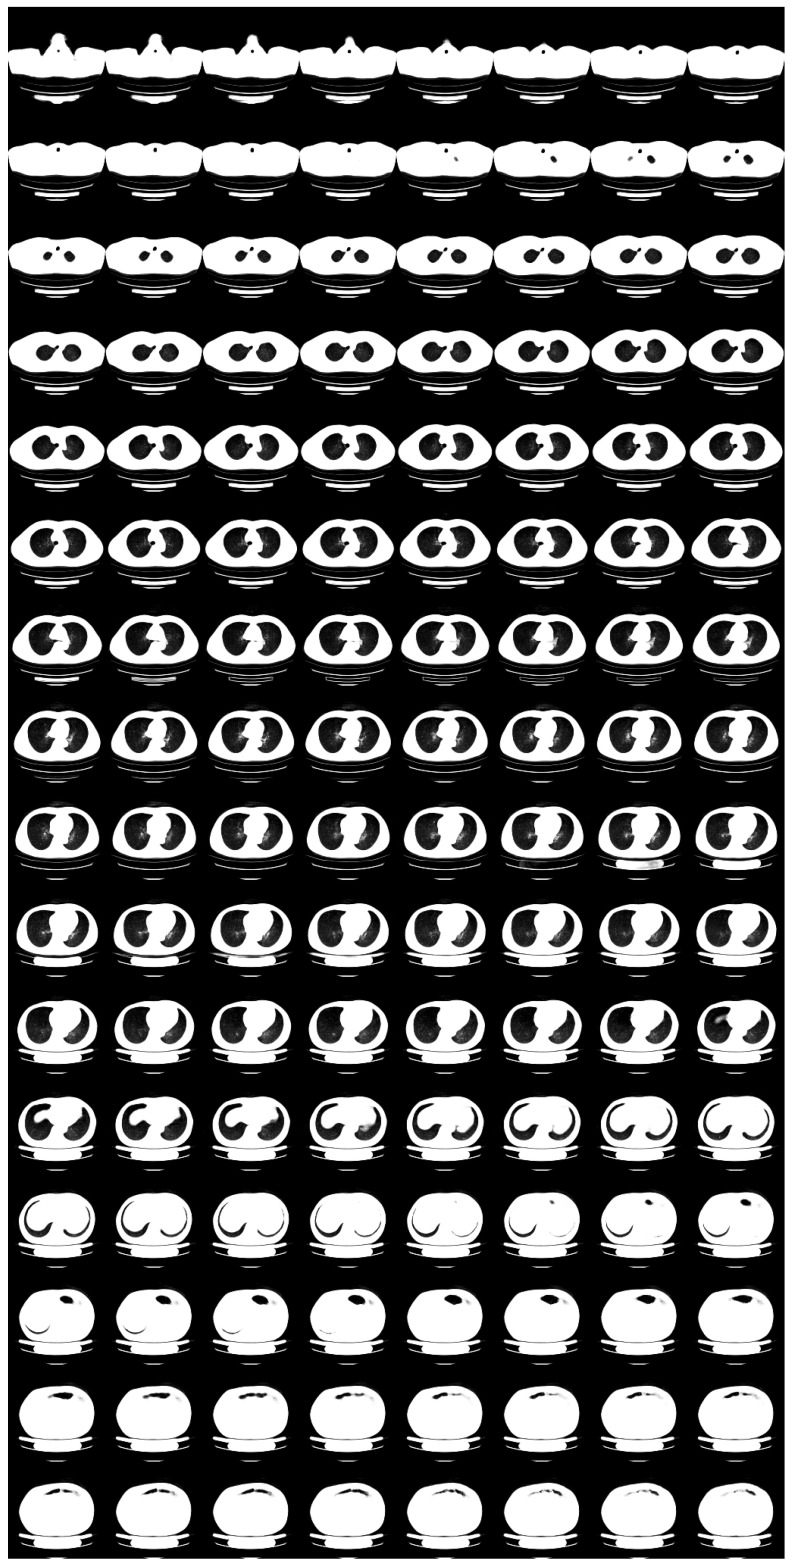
Reconstructed 3D CT image with X2CT-FLOW from Figure 3a,b ($\sigma^{2}=0,N=2$), in pulmonary window setting.
